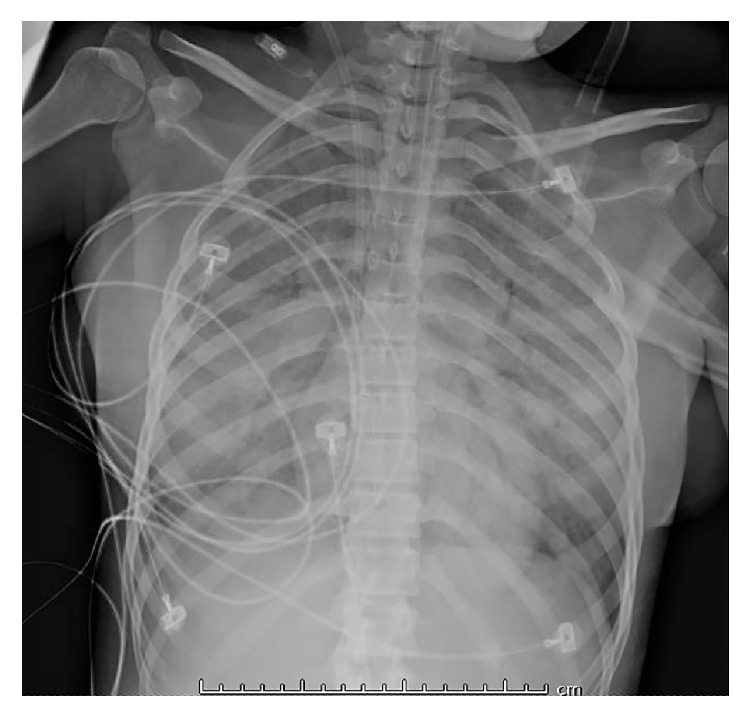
Chest radiograph showing diffuse dense bilateral airspace opacification with lower lobe predominance compatible with pulmonary hemorrhage/edema.
